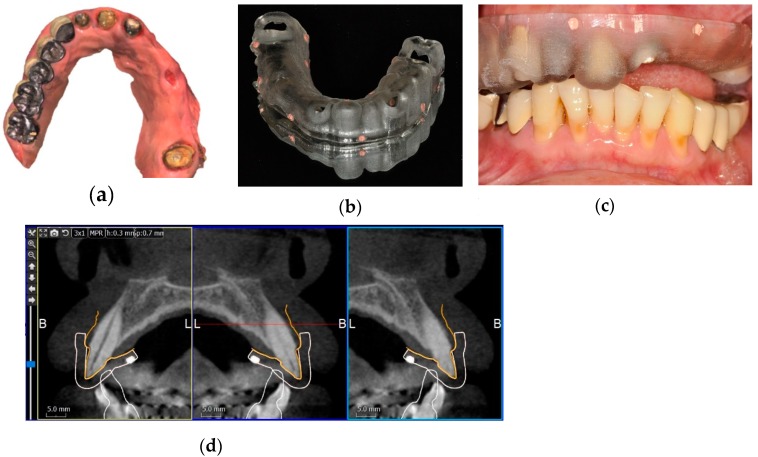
Fiducial marker protocol of implant planning. (a) An occlusal image of a maxillary intraoral scan. Five metal crowns on the right side and the inadequate number of residual teeth made the correct alignment of CBCT and intraoral scans by dental surface registration difficult. (b) Using the digital data of the intraoral scan, a stereolithographic (SLA) radiographic template with gutta-percha fiducial markers was fabricated. (c) The SLA radiographic template in the mouth ready for a CBCT scan. (d) A CBCT image aligned with the intraoral scan image (orange line) by matching the fiducial markers (white cubes) on the SLA radiographic template (white line).
